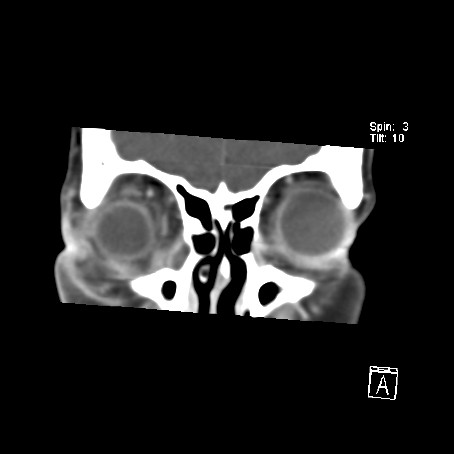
CT scan of the orbits and brain, coronal view. Soft tissue density collection on the floor of the right orbit, elevating the inferior rectus muscle, extending posteriorly to the apex of the orbit.
